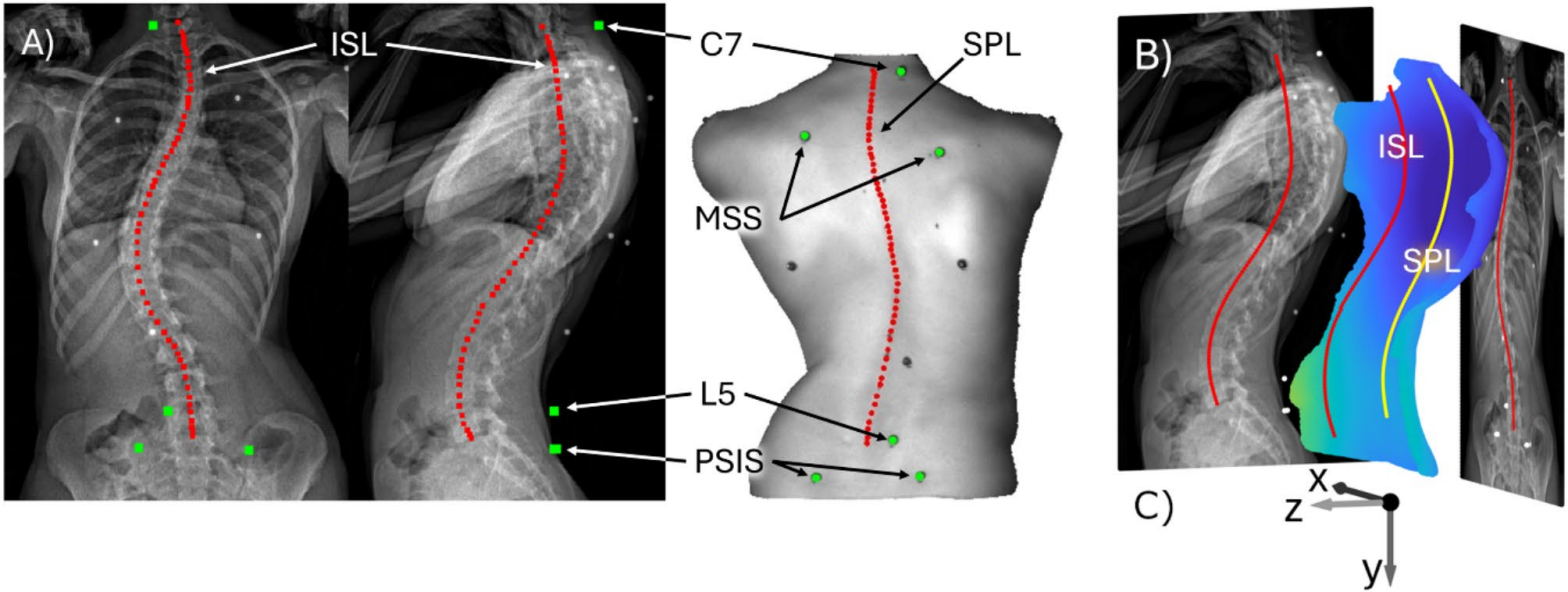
(A) Manual selection of anatomical landmarks C7, MSS, L5, PSIS, SPL, and ISL with (B) automatic registration of the 3D back surface scan with the biplanar radiographic images in PCdare, and (C) coordinate system (x, y, z).
